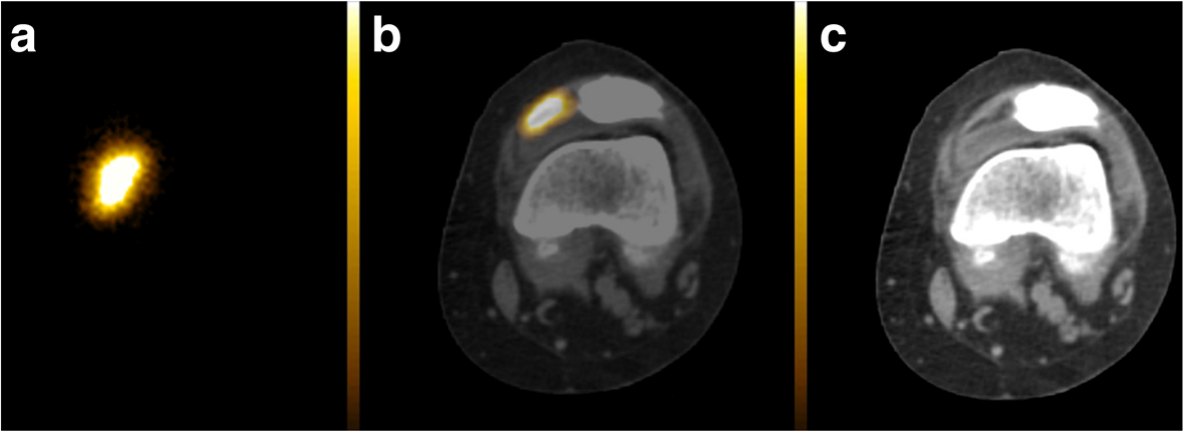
Anterior bremsstrahlung planar (a), axial fused PET/CT (b) and co-registered CT (c) images of the left knee demonstrating a focal (non-diffuse) planar imaging Y-90 pattern located extra-articularly on fused PET/CT (superficial to the deepest capsular layer but deep to the medial patellar retinaculum)
